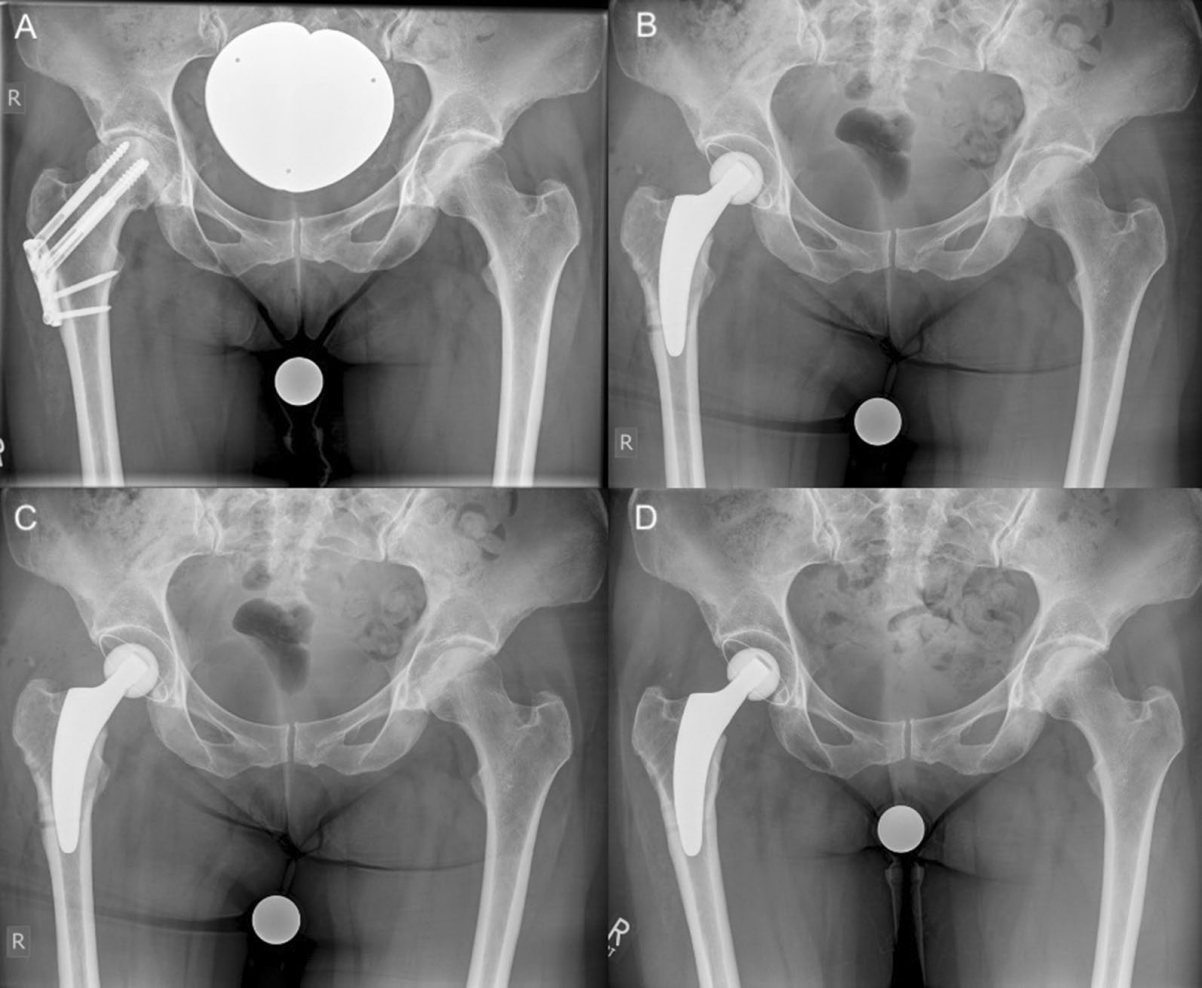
Radiographs of a 53-year-old female patient with secondary osteoarthritis: A preoperative; B postoperative; C 6-week follow-up D: 26-month follow-up (no signs of loosening or fracture)
